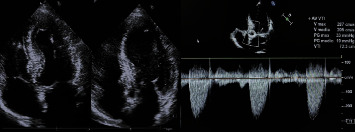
Echocardiographic evaluation at 12 months.
